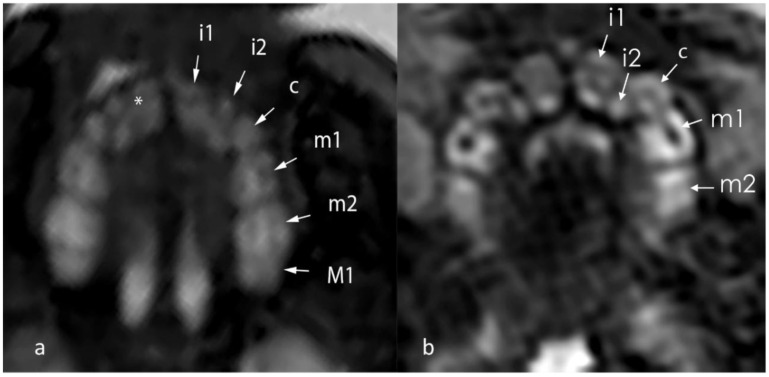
T2-weighted ssFSE axial images of two GW 36 fetus, performed at 1.5T (a) and 3T (b) displaying the tooth buds: i1 (first primary incisivus), i2 (second primary incisivus), c (primary caninus), m1 (first primary molar), m2 (second primary molar), M1 (first permanent molar). The asterisk shows the calcified edge within the tooth bud.
